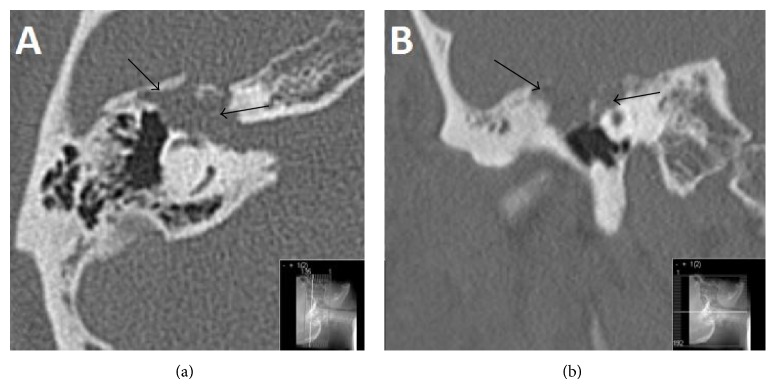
Axial (a) and coronal (b) CT images demonstrating a destructive abnormality (*black arrows demarcating extent*) involving the right geniculate ganglion extending into the right attic and eroding the bone around the right cochlea.
